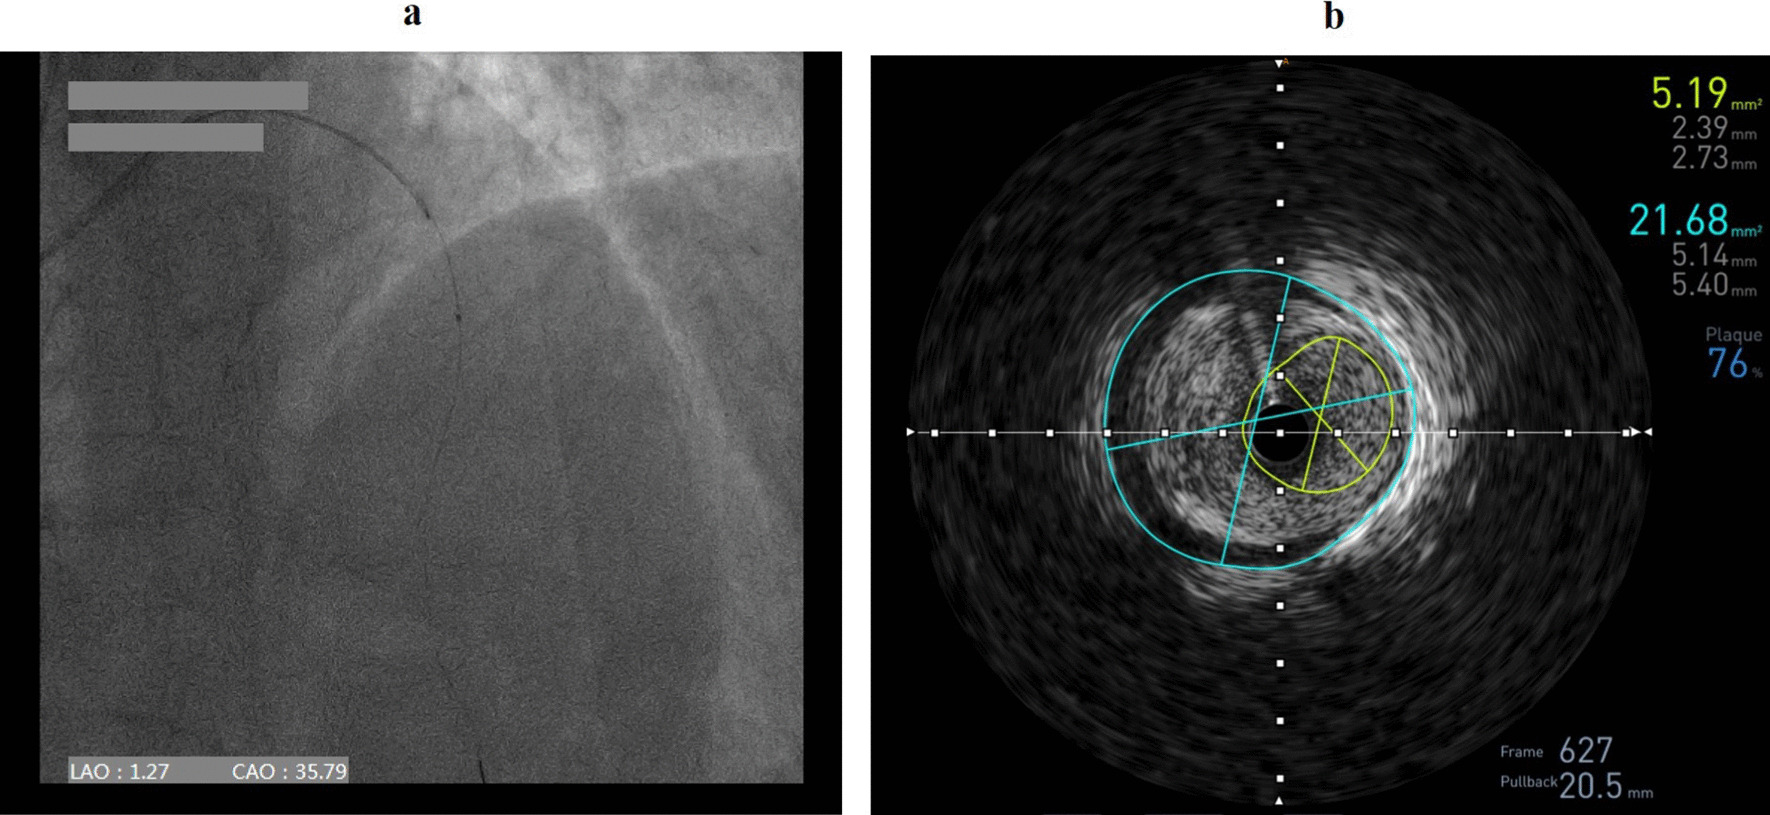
Intravenous ultrasound images. a Intravenous ultrasound (IVUS) images were acquired by automated mechanical pullback devices with a continuous pull back speed of 0.5 mm/s. b The lumen area of the LAD lesion was 5.19 mm^2^ and the plaque load was 76%
